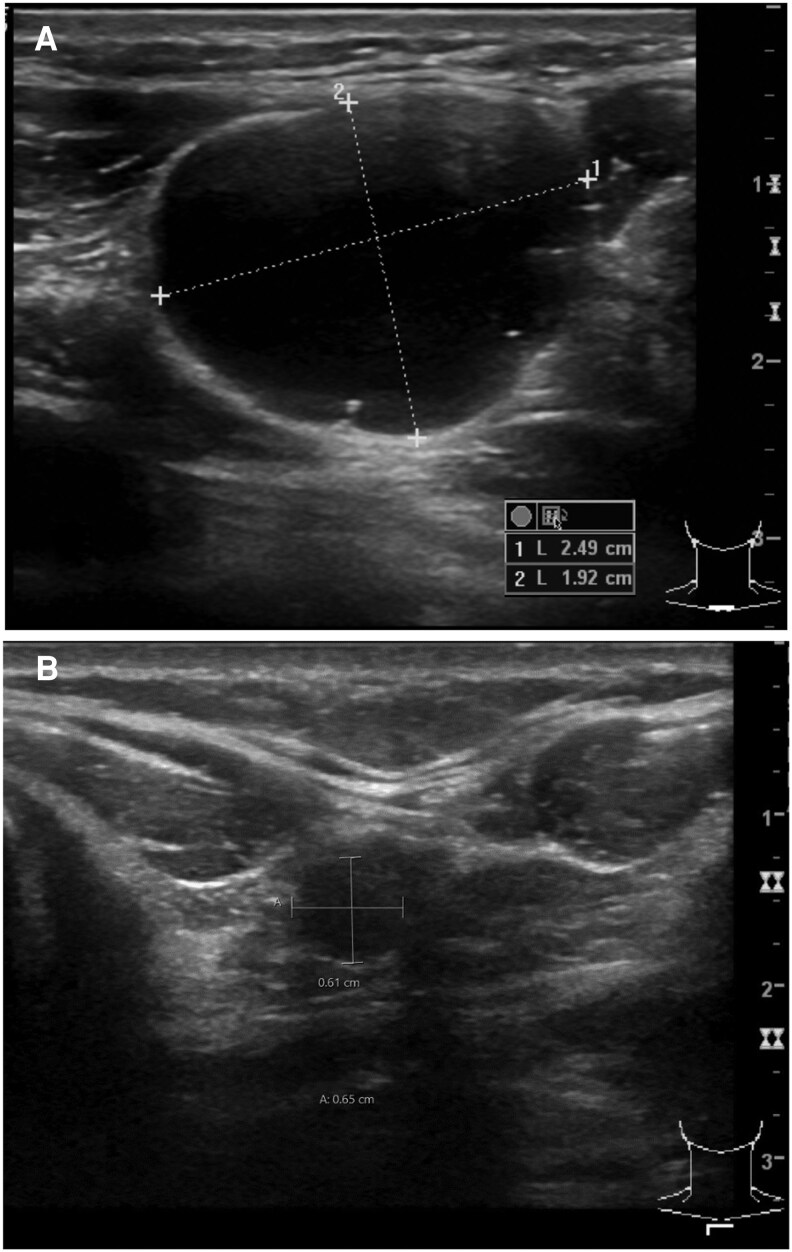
A, A large pure cystic metastatic lymph node (MLN) of papillary thyroid carcinoma in a 37-year-old male patient (T2N1bM0, stage I), measured 25 × 19 × 21 mm (5.2 mL) before ethanol ablation (EA). Initially, the MLN was treated using an injection of 0.6 mL of ethanol. B, Six months later ultrasound (US) examination showed a reduction in size to 6 × 7 × 7 mm (0.15 mL). A second EA was performed, this time injecting a total of 0.3 mL of ethanol. No residual lesion was seen in the following US. Two noncystic MLNs in the same region were also treated by EA; however, neither exhibited a primary response after 9 and 1 injections, respectively. The large cystic MLN (A and B) remained undetectable until it was lost to follow-up 3 years after the initial EA, at which point a neck node dissection was performed to remove the 2 nonresponsive MLNs in the same area. The patient had loco-regional control with no detectable MLN in the neck at follow-up examination 11 years (133 months) after primary surgery.
